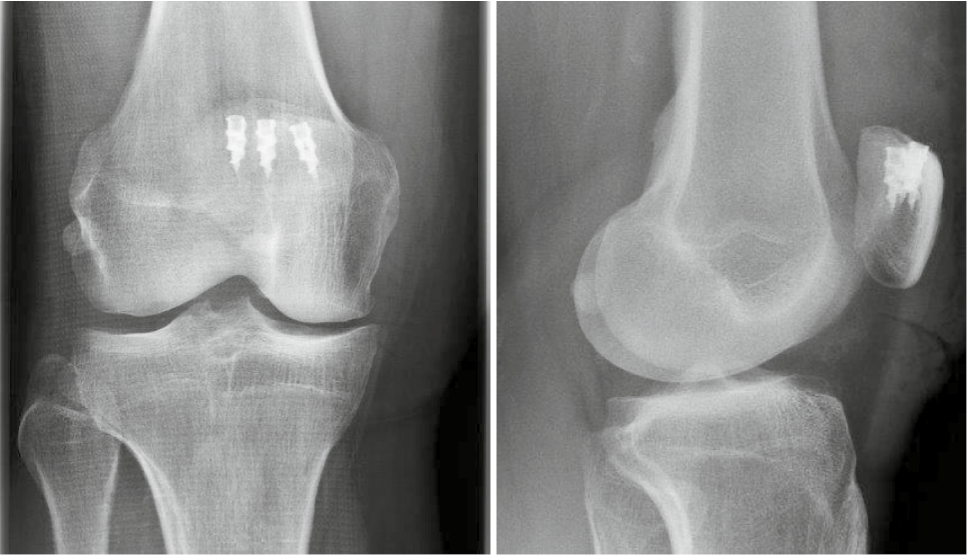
Postoperative radiographs. The antero-posterior and lateral *X*-ray of the right knee show correct anchor placement at the superior margin of the patella with three double-loaded 5.5 mm titanium suture anchors (Corkscrew^®^, Arthrex, Naples, USA)
